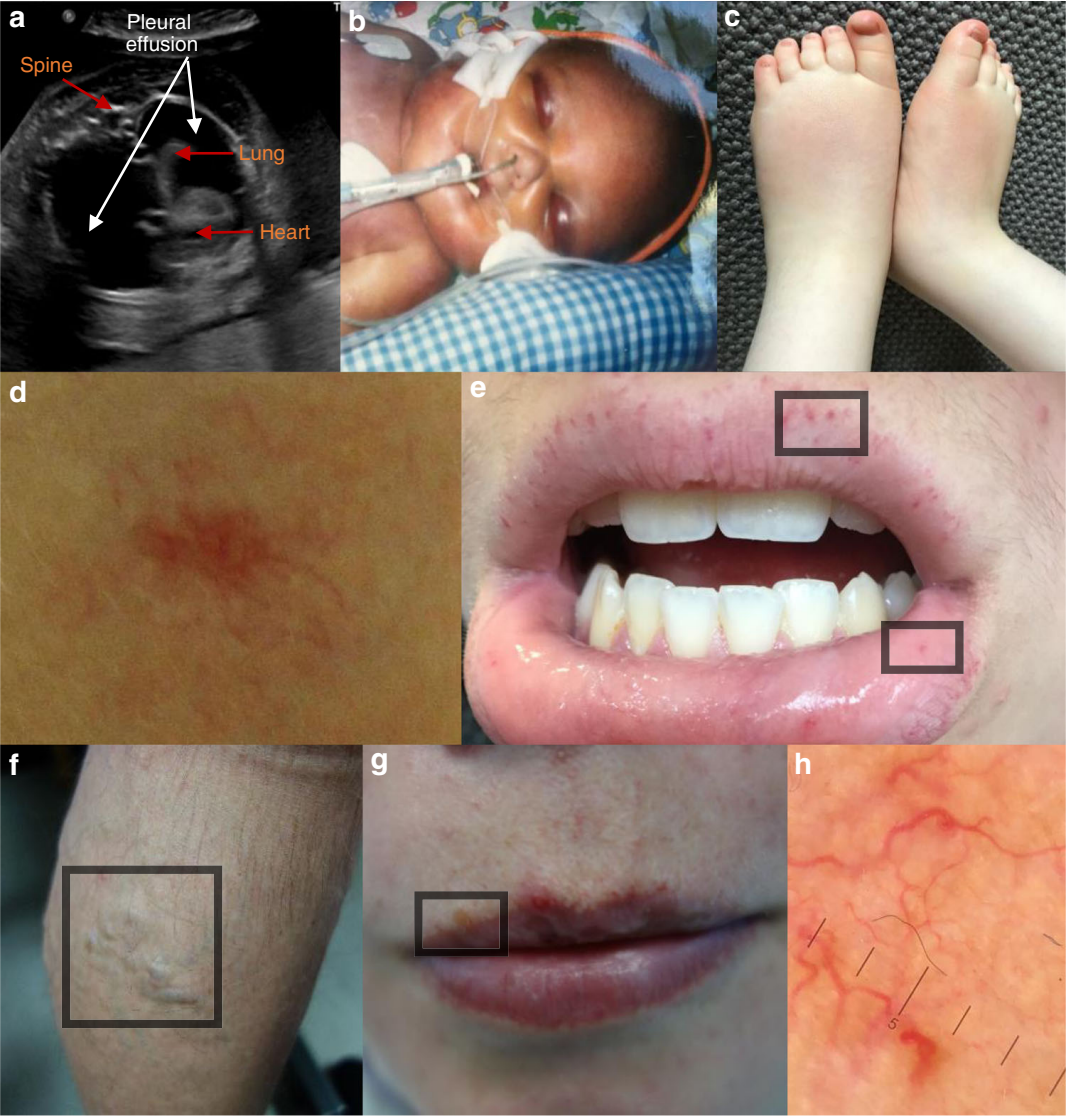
Clinical findings in individuals with *EPHB4* variants. (a) Antenatal ultrasound scan (transverse plane) demonstrating bilateral pleural effusions at gestational week 30 + 4 in FH2:II.1. (b) Baby in the neonatal period with fetal hydrops (GLDUK:II.6). (c) Persistent peripheral lymphedema in the feet of FH5:II.2 at age 4 years. (d) Capillary malformation in the midline of the neck in VA1:II.2. (e) Multiple telangiectasia along the vermilion border of the upper lip and the mucous membrane of the lower lip in VA2:II.1 (inside boxed areas). (f) Early onset and extensive lower limb varicose veins in GLDUK:I.2. (g) GLDUK:II.4 with multiple telangiectasia with a propensity for the vermilion border of the lips (inside boxed area). (h) Dermatoscopic image of telangiectasia on the left cheek confirming the presence of dilated linear and branching capillary vessels in GLDUK:II.4.
